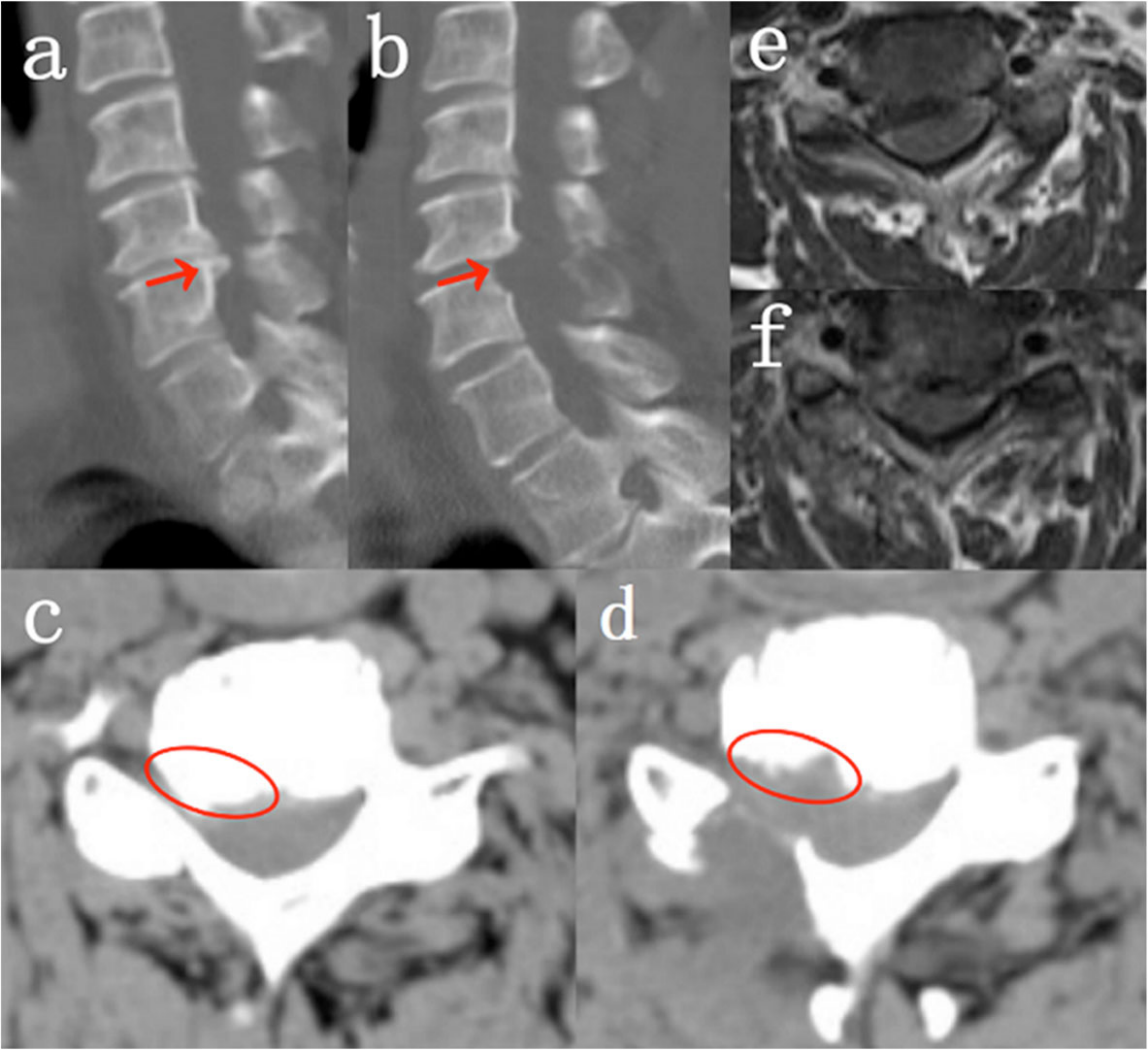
Preoperative and postoperative radiological characteristics of PPECD-VBD. a Preoperative CT sagittal image.b Postoperative CT sagittal image. c Preoperative CT axial image of the surgical segment. d Postperative CT axial image of the surgical segment. e Preoperative MRI axial image of the surgical segment. f Postperative MRI axial image of the surgical segment
